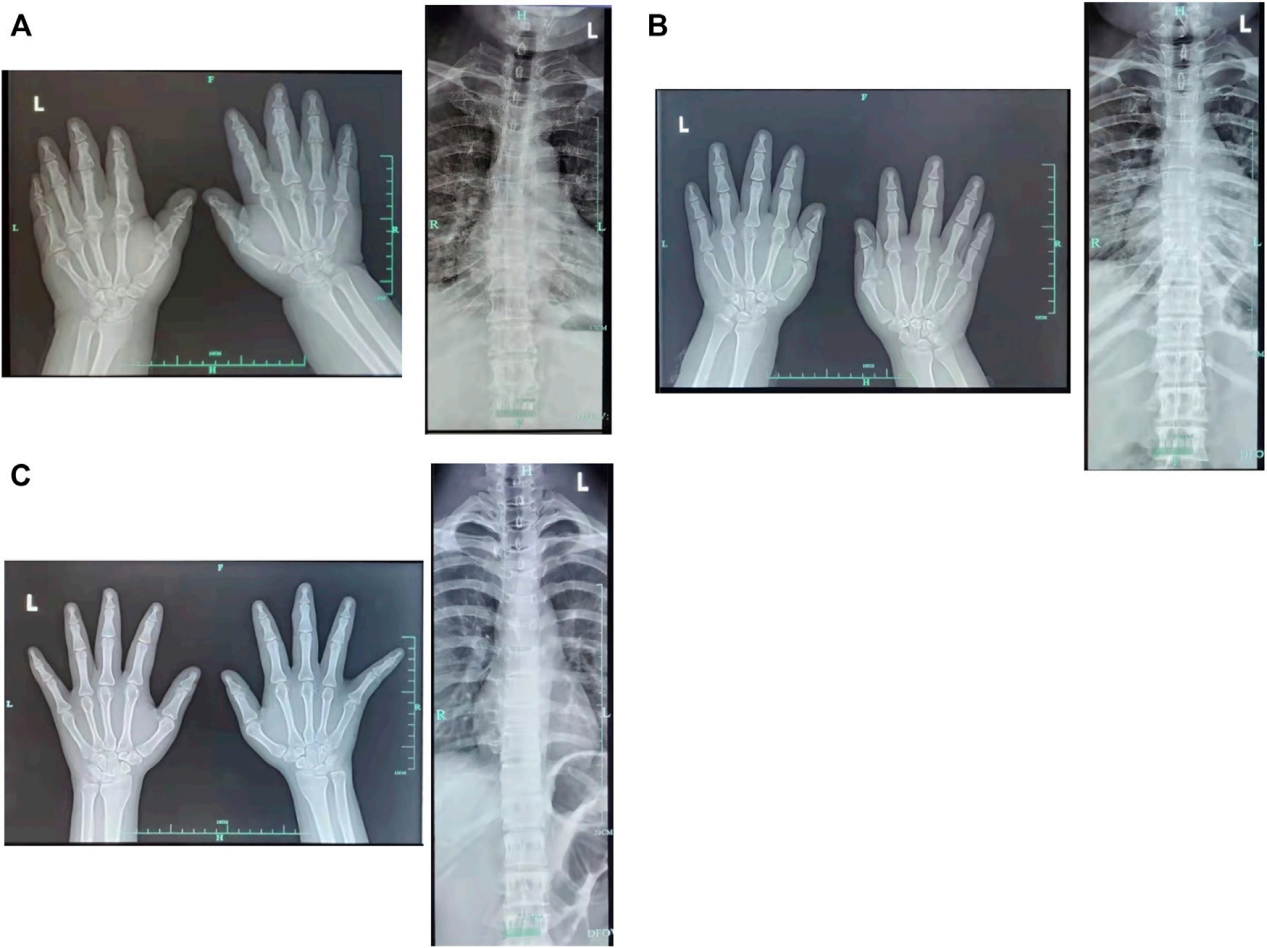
X-rays of hands and spines. (A) Mother (B) Elder sister (C) Proband.
